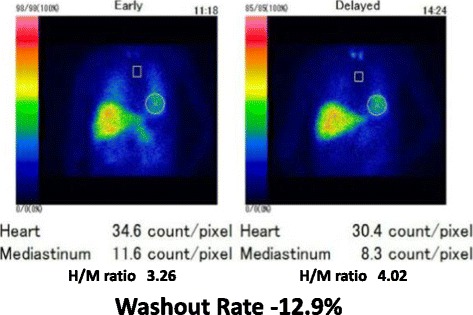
Cardiac ^123^I-meta-iodobenzylguanidine (MIBG) scintigraphy results in 2015. The values of the heart to mediastinum (H/M) ratio in the early and delayed phases were 3.26 and 4.02 (normal range is > 2.20), respectively. The washout rate was − 12.9% (normal range is < 22%). ^123^I-MIBG scintigraphy showed normal cardiac MIBG uptake
